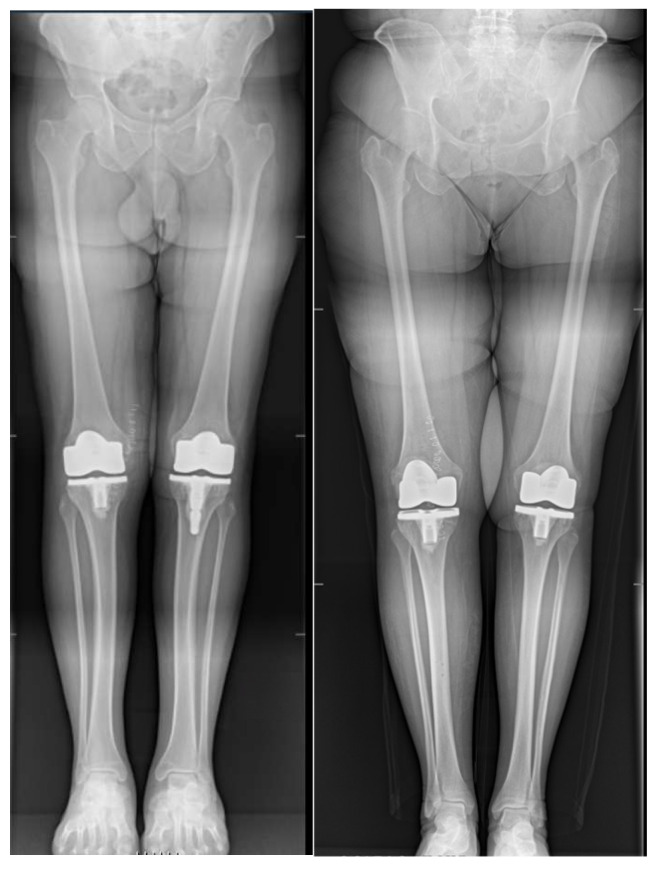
Example of a full-length weight-bearing anteroposterior lower limb radiograph used for measurements.
